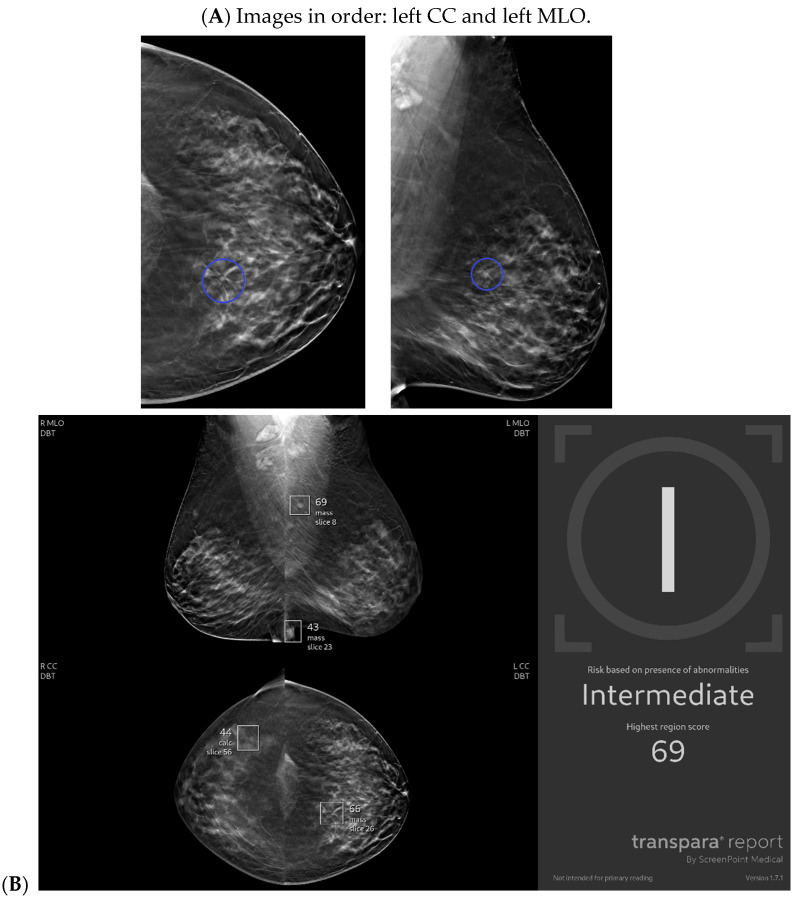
New architectural distortion detected by artificial intelligence (AI): A patient in her 50s’ screening mammogram revealed a new area of architectural distortion (circle) in the inner central region of the left breast (A). The AI program Transpara highlighted potential regions of interest, including this suspicious area of architectural distortion on the left breast on the corresponding left CC view; however, AI also highlighted benign areas that were arbitrated out by the radiologist (B). There was no sonographic correlate, so a stereotactic biopsy of this area of architectural distortion was then biopsied under guidance. Pathology yielded invasive lobular carcinoma. Images obtained from the Icahn School of Medicine at Mount Sinai.
